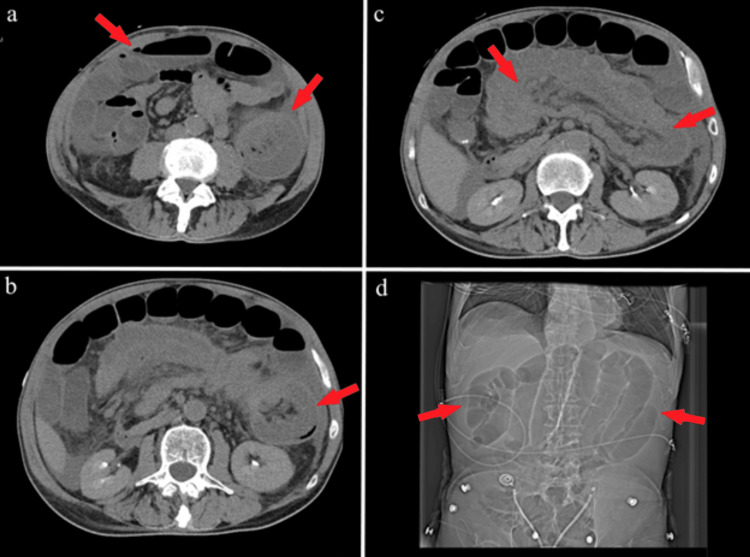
Preoperative CT imaging. (a) Left arrow: dilated bowel loops along with an air-fluid level concerning for small bowel obstruction; right arrow: mesenteric swirl raising the possibility of volvulus. (b) “Target” sign of bowel within bowel indicating possible intussusception. (c) Long section of mesenteric fat along a segment of intussusception. (d) Topogram showing multiple dilated loops of small bowel.
